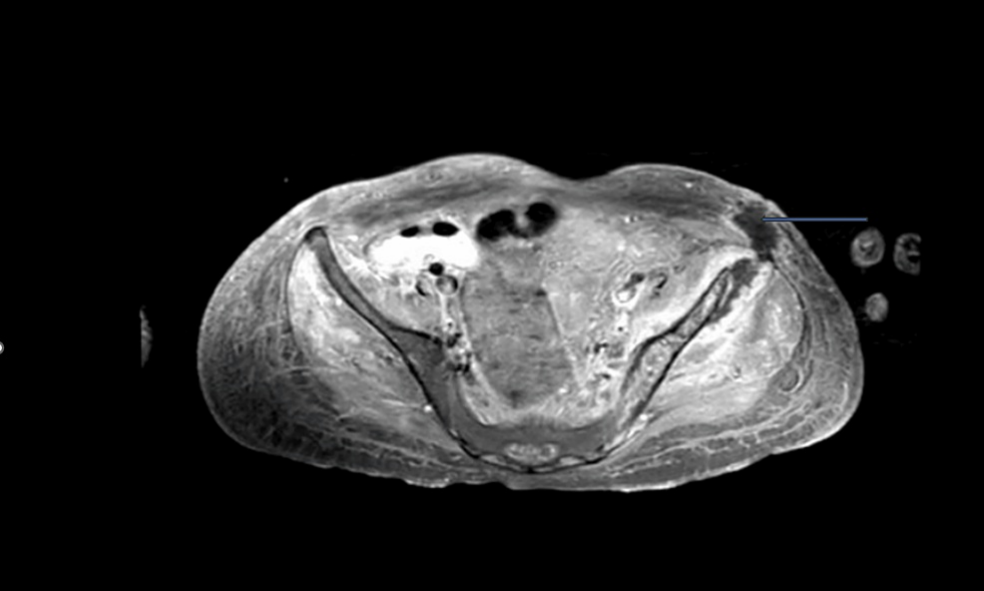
Magnetic resonance imaging of the pelvis The blue arrow shows the left gluteal abscess.
